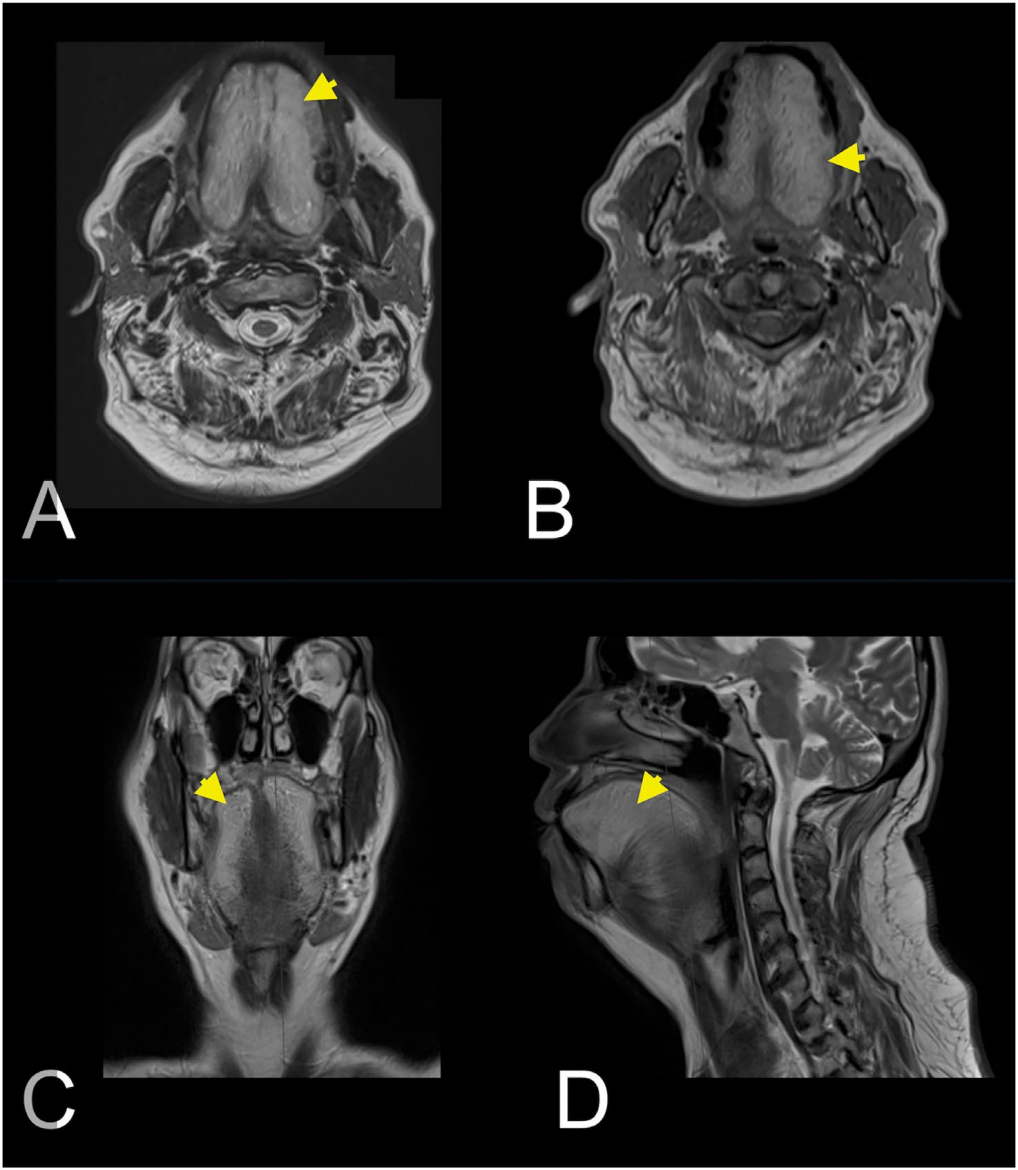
(A) Axial T2‐weighted MR image showing benign symmetrical lipomatosis (BSL) with high signal intensity (arrowheads), typical of adipose tissue. (B) Axial T1‐weighted MR image confirming BSL with a similar high signal intensity pattern. (C) Coronal T2‐weighted MR image showing BSL with high signal intensity (arrowheads). (D) Sagittal T2‐weighted MR image demonstrating BSL with high signal intensity (arrowheads).
